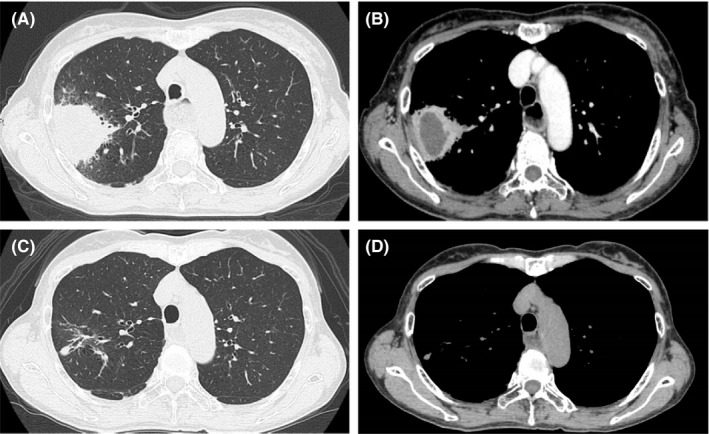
(A,B). Computed tomography (CT) of the chest showed a 50 mm abscess with air‐fluid levels in the right upper lung lobe. CT also showed dilatation of the esophagus, suggestive of esophageal achalasia. (C,D) Follow‐up CT showed resolution of the lung abscess
